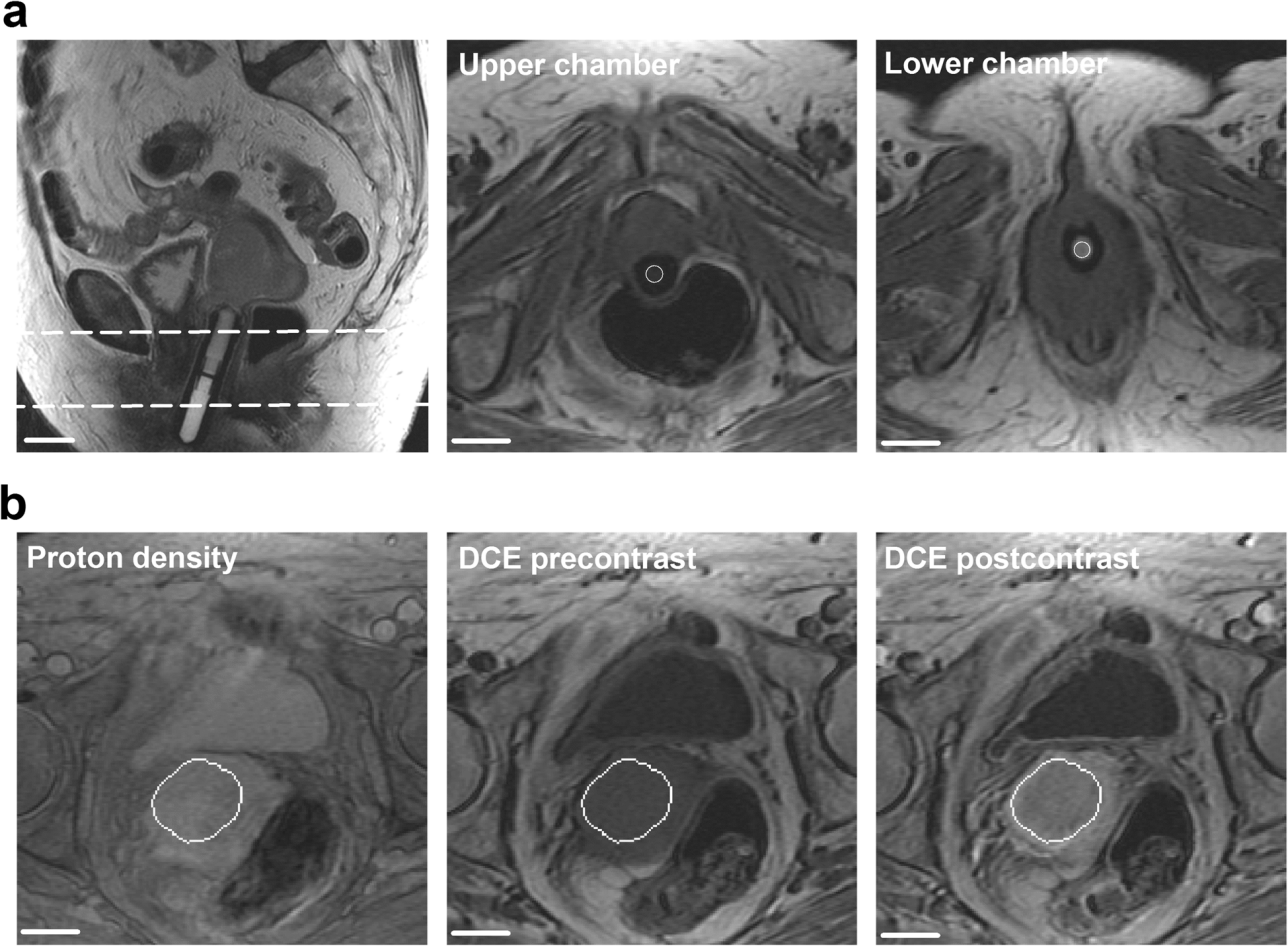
Representative anatomical MR images. a A sagittal and two axial *T*_1_-weighted scans showing the signal intensities of the two-chamber calibration tube. The dashed horizontal lines indicate the positions of the axial scans. b A proton density-weighted image, a precontrast *T*_1_-weighted image, and a postcontrast *T*_1_-weighted image showing the signal intensities of the tumor tissue. Scale bars: 2 cm
